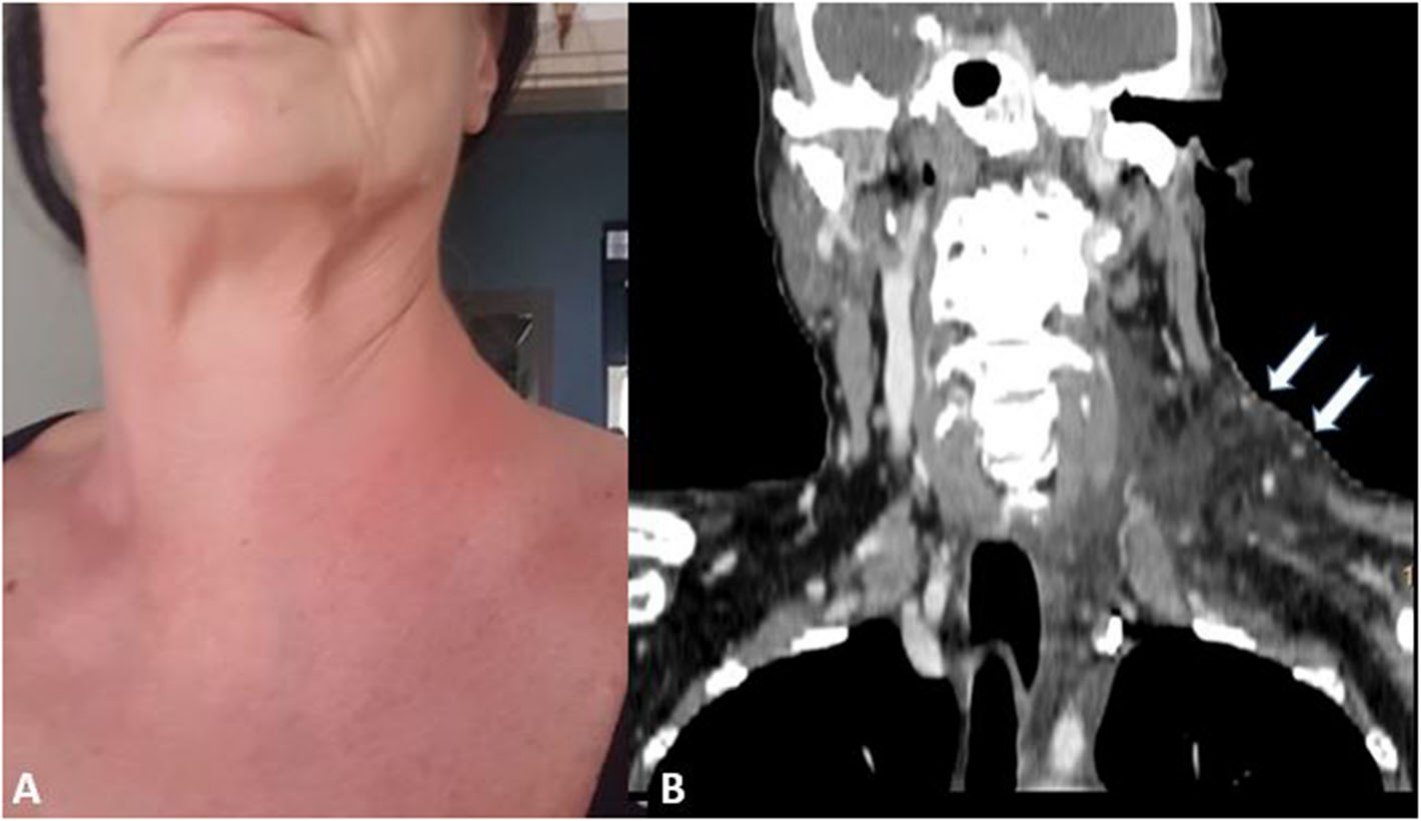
A 64-year-old woman with a sudden left lateral cervical swelling (A) and the corresponding coronal multiplanar reconstruction (MPR) contrast-enhanced CT images at the admission (B) showing the soft-tissue swelling (white arrows)
